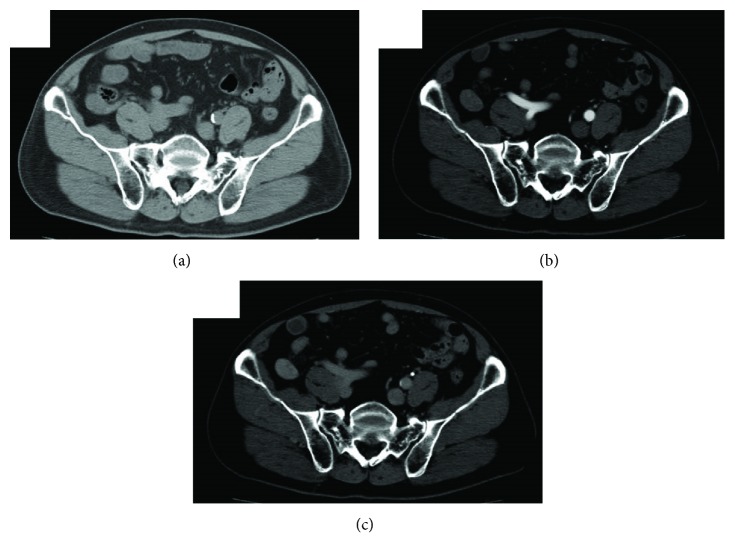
Abdominal CT showed well-defined isodensity oval mass at the tip of appendix (a). In contrast-enhanced CT, the lesion was gradually enhanced homogeneously from arterial phase (b) to equilibrium phase (c).
